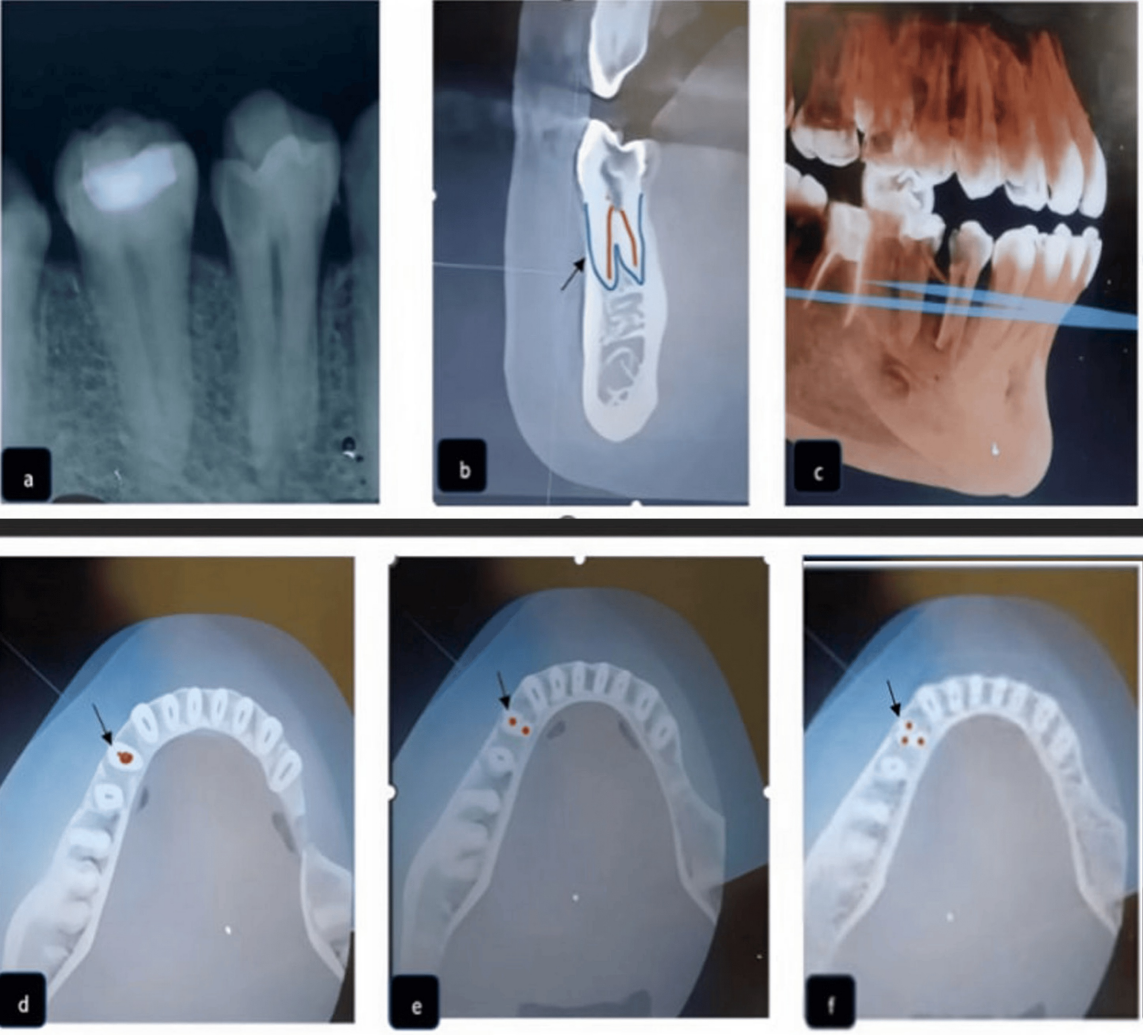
Pre-operative radiographic and CBCT images. (a) Pre-operative radiograph; (b, c) pre-operative CBCT sagittal cross-sections, here the blue line represents the root border and the red line represents the canal configuration; (d) axial view at the coronal third; (e) axial view at the middle third; (f) axial view at the apical third. In (d-f), red dots represent the canal configuration, and arrows pointing to the dots indicate the labial side. CBCT: cone beam computed tomography
